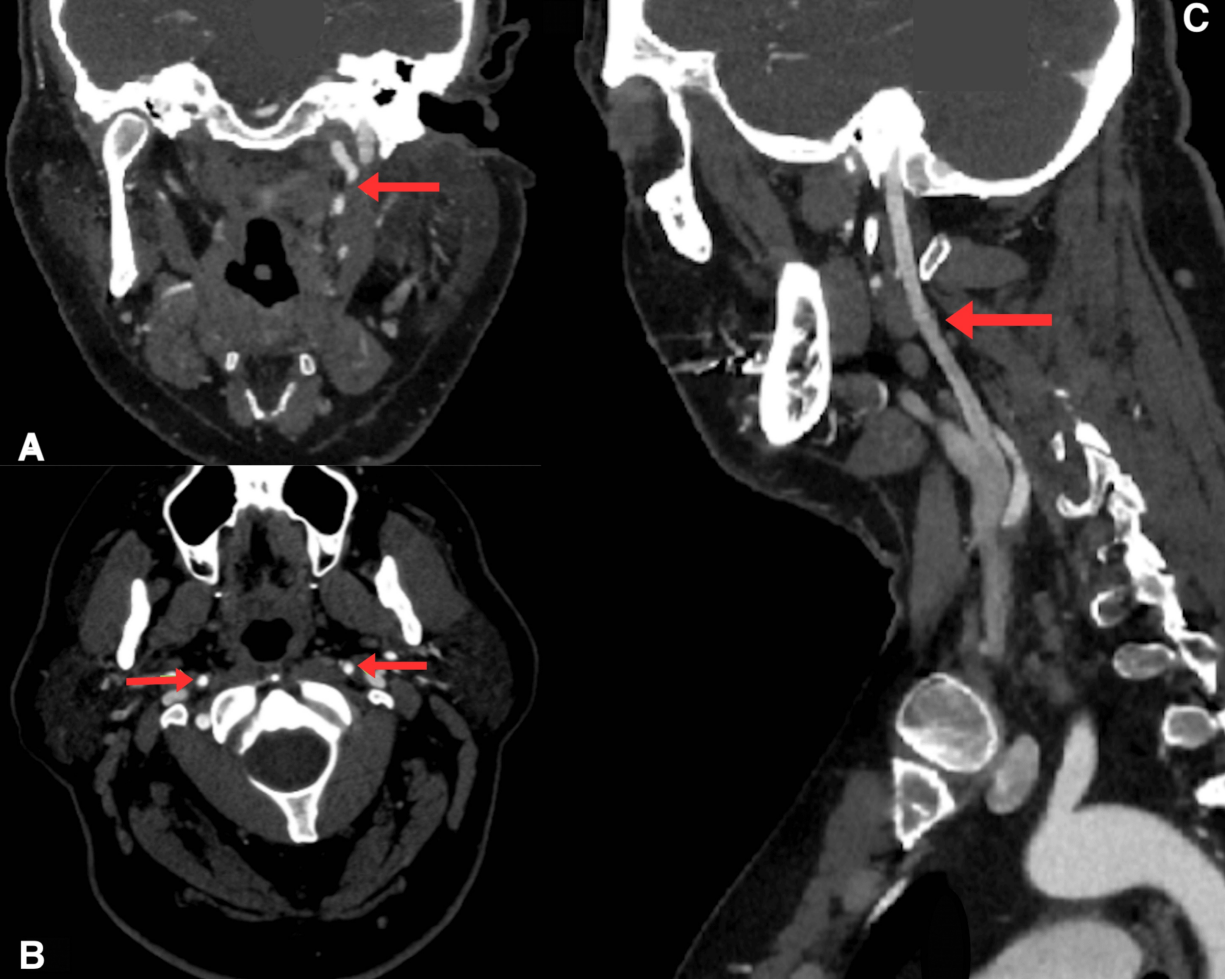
CT angiography demonstrating recanalisation of the ICA following dissection, observed at six-month follow-up (A) Coronal CT angiography showing recanalisation of the left ICA, (B) axial slice demonstrating the right ICA with recanalisation of the left ICA with symmetrical blood flow in the ICA, and (C) sagittal view showing left ICA recanalisation
